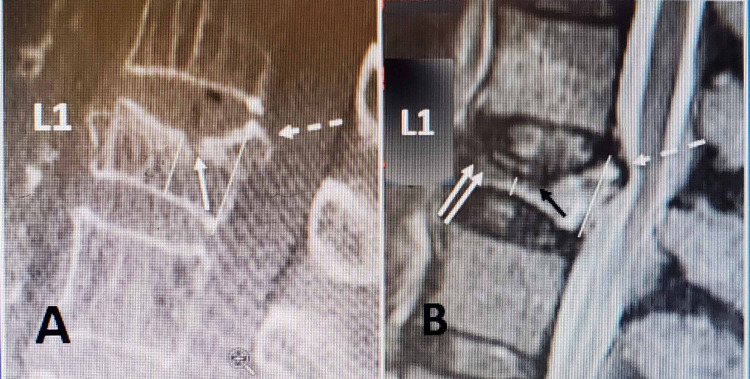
Patient with an acute L1 fracture seen on MRI and compared with bone detail seen on CT scan. CT demonstrates that fracture has progressed in less than four weeks to a vertebra plana. A: CT scan performed two weeks after fall of a L1 fracture showing fracture in middle and slightly posterior superior endplate (solid white arrow). There is a 3.5 mm posteriorly displaced superior endplate (dashed white arrow). The degree of collapse is approximately 50% indicated by thin solid white arrows posteriorly and in middle of the collapsed vertebra. B: MRI scan on same patient after being seen four weeks later compared to CT scan. The collapse has progressed to 80% (small solid white arrows), and the fracture of the superior endplate (double white arrows) has become more severe in the middle and anterior areas. The solid black arrow shows the progressive mid-vertebral collapse that occurred in the interval from CT to MRI scan. The posteriorly displaced superior endplate is now over 5 mm in size and extends more inferiorly compared to what was seen on CT scan (dashed white arrow) and is involving the middle of the posterior edge of vertebra wall abutting the spinal canal. CT, Computerized tomography; MRI, magnetic resonance imaging.
